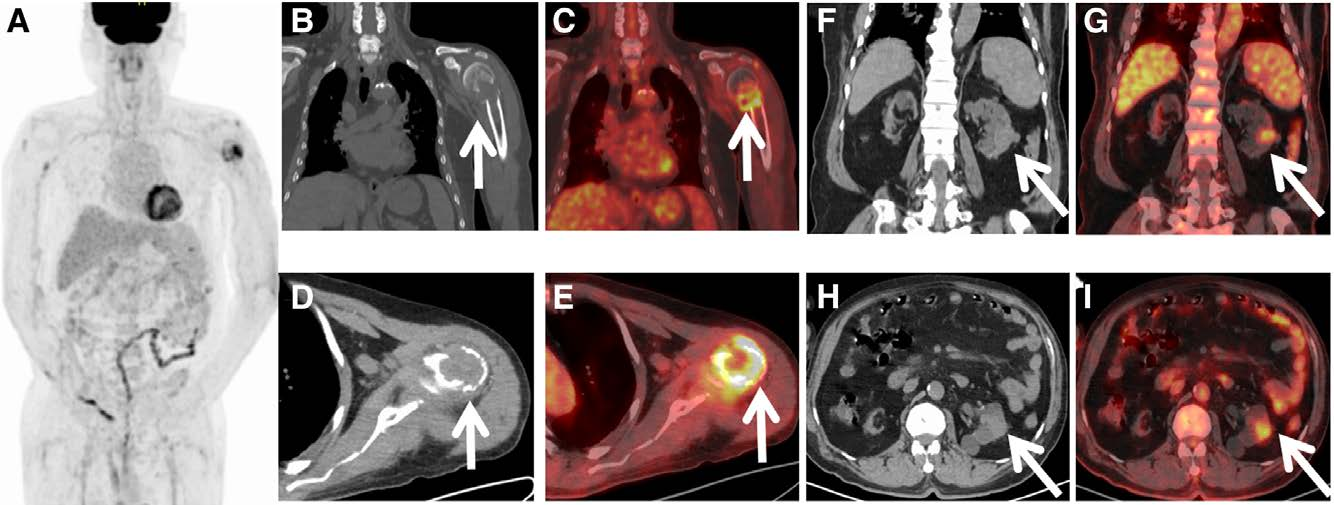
The patient underwent an ^18^F-FDG PET/CT scan based on suspected malignant tumor. The MIP (A) images show a high ^18^F-FDG uptake in the left humerus. Coronal (B) and axial (D) CT images show bone destruction and soft tissue formation in the humerus, with fractures (arrows). The PET/CT fusion images (C, E) show high uptake of ^18^F-FDG (SUVmax, 8.6). Coronal (F) and axial (H) CT images show a soft tissue lesion in the lower part of the left kidney. PET/CT fusion images (G, I) show moderate uptake of ^18^F-FDG (SUVmax, 3.1). ^18^F-FDG PET/CT = ^18^F-fluorodeoxyglucose positron emission tomography/computed tomography, CT = computed tomography, MIP = maximum intensity projection.
